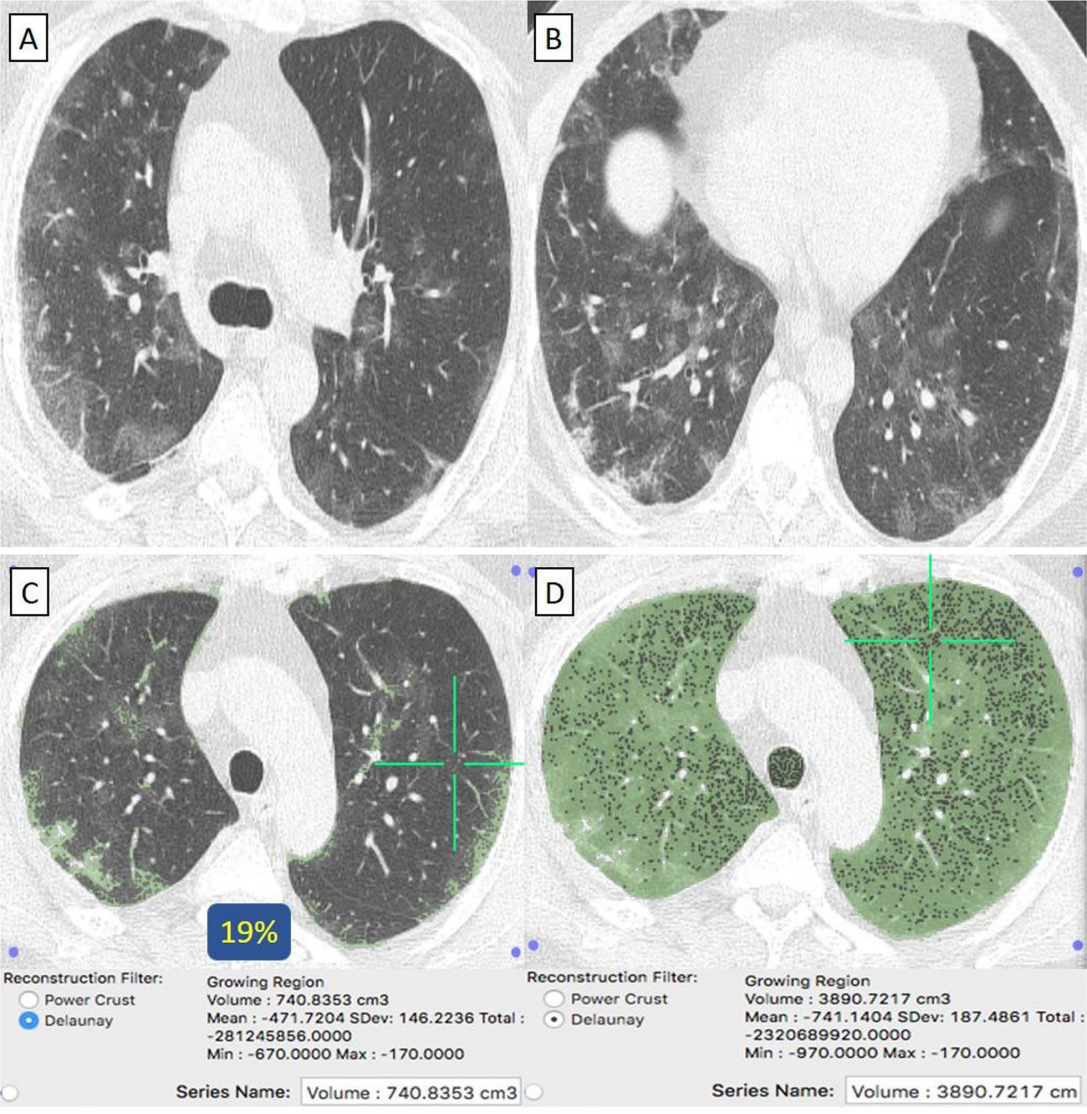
A 58-year-old female patient with negative past medical history and had received “Sinopharm” vaccine then got COVID-19 infection. A, B Axial chest CT cuts (lung window) showed bilateral mainly peripheral located ground-glass pneumonic patches and minimal right pleural reaction. C CT-Volumetry of the pathological lung parts averages 740 cc. D CT-Volumetry of total lung volume averages 3890 cc. So, only 19% of the lung parenchyma was diseased (Score 1)
